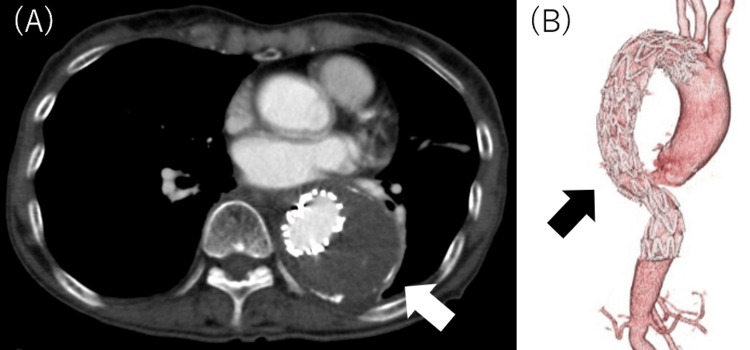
Postoperative contrast-enhanced CT. (A) No endoleak was observed on the short-axis image (white arrow). (B) A 3D reconstructed image. The stent graft was deployed distal to the left subclavian artery bifurcation and proximal to the celiac artery bifurcation (black arrow). The image is visualized from a dorsal perspective.
